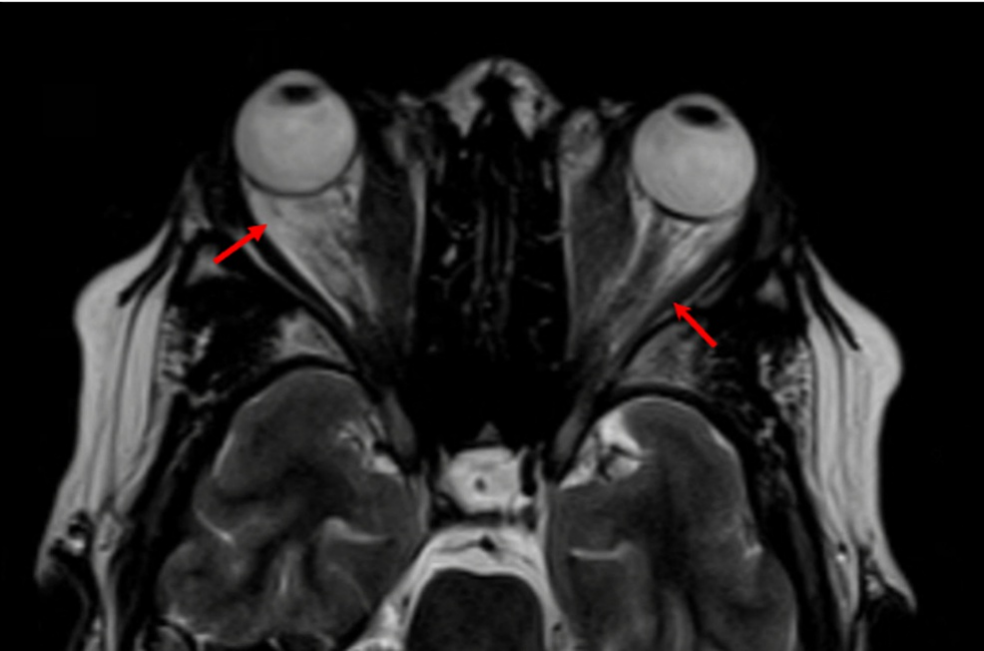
Orbital MRI (axial view) showing bilateral proptosis. There is a generalized increase in the volume of orbital fat (indicated by red arrows). MRI, magnetic resonance imaging
